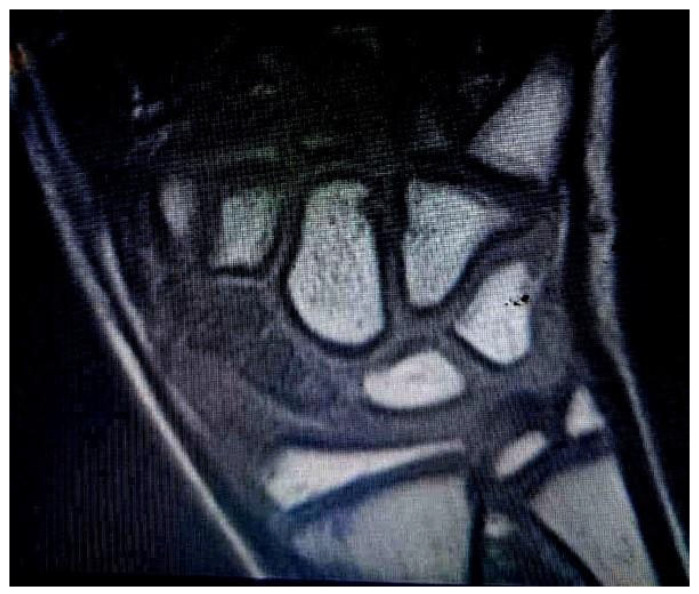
Vascular damage to the whole scaphoid determined via MRI (in the T1 TSE STIR COR sequence) in Patient 1.
